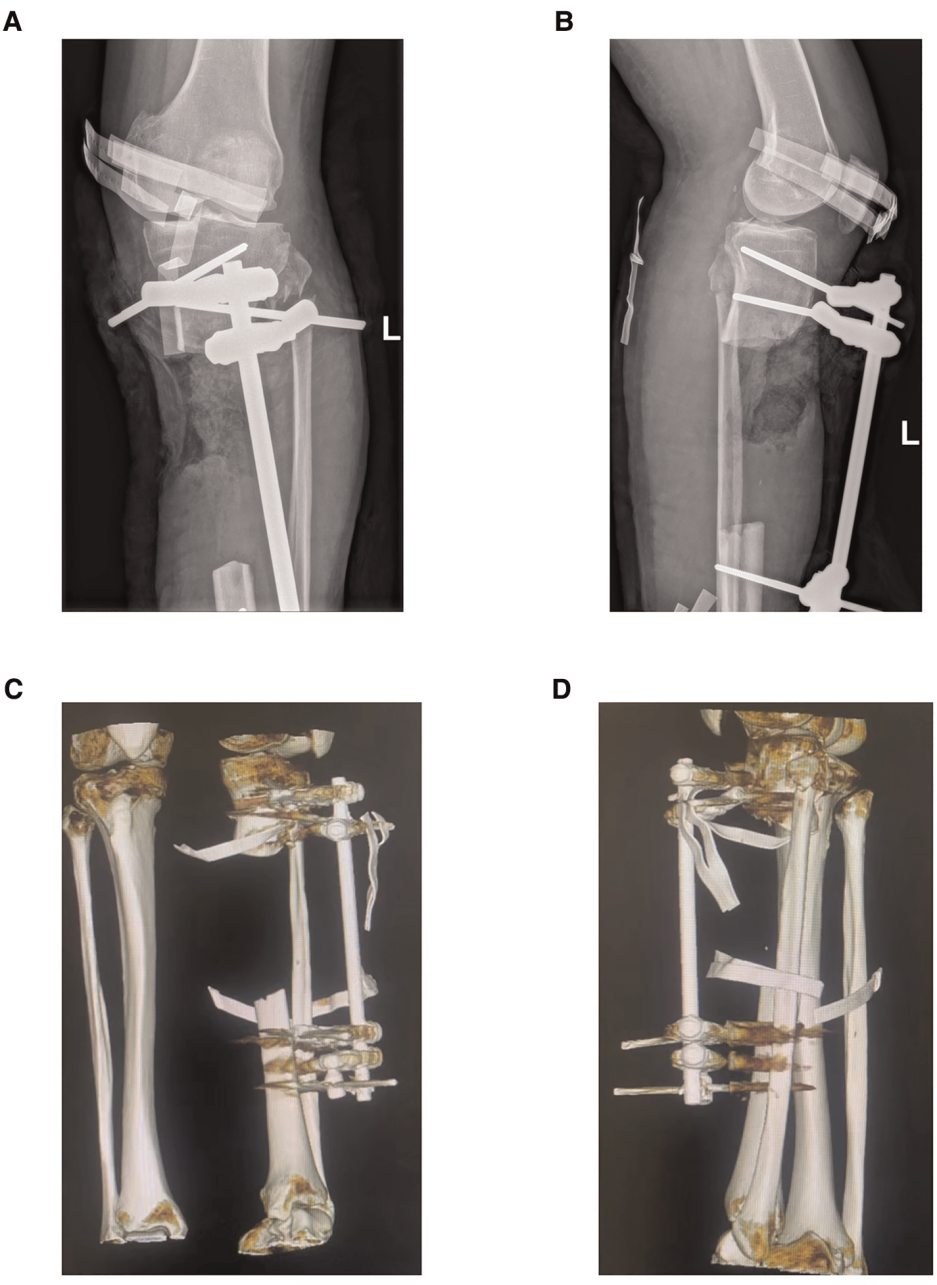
Preoperative x-ray and three-dimensional reconstruction of computed tomography (CT) of the left lower limb in case 1. (A) Preoperative x-ray on anterior-posterior (AP) view of affected limb in case 1, and there is an approximately 144 mm long bone defect at the proximal tibia. (B) Preoperative x-ray on lateral view of affected limb in case 1. (C,D) Three-dimensional reconstruction image of case 1 before operation.
